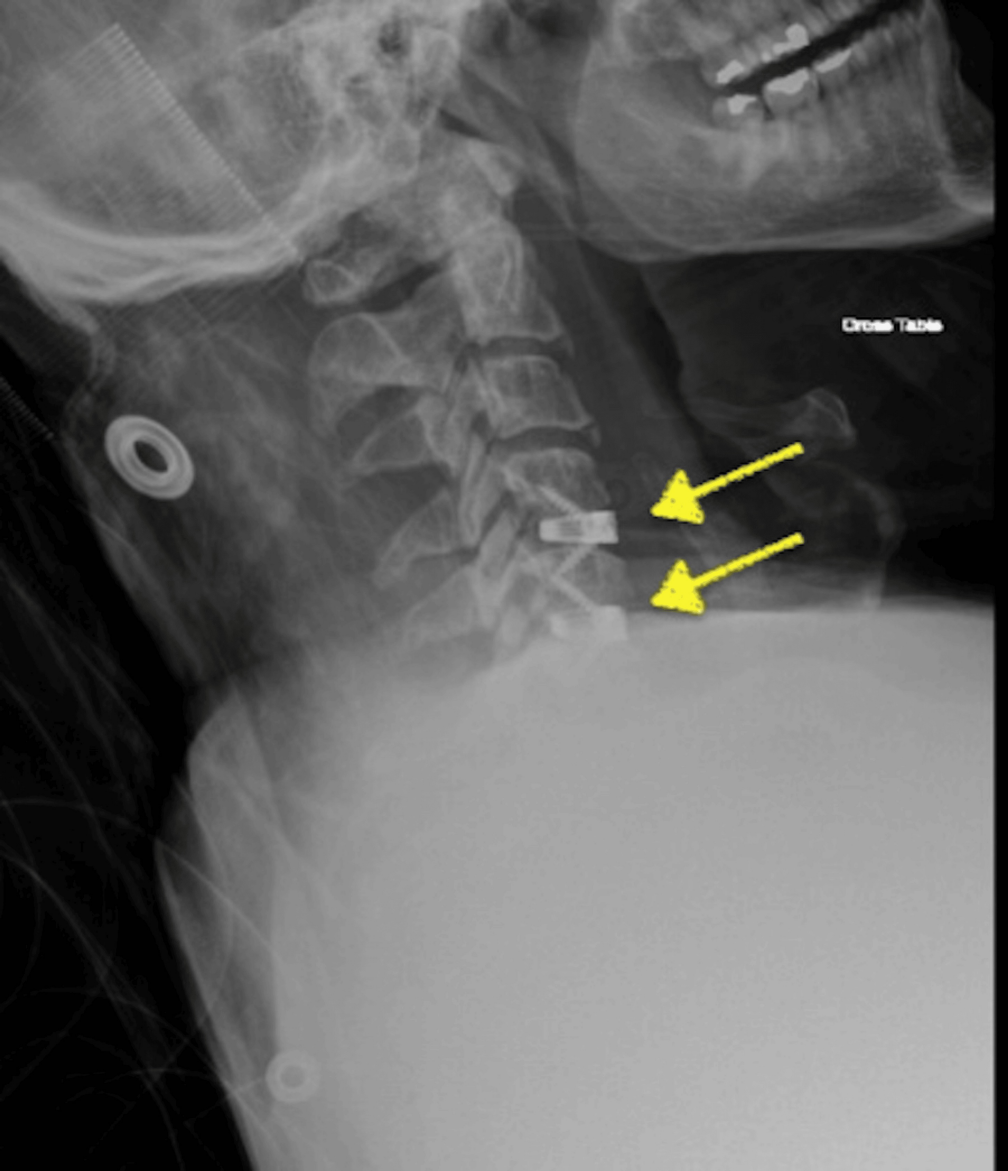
X-ray with lateral view of the cervical spine confirming postoperative proper screw placement and inter body spacers (yellow arrows) at C4-C6 levels.
